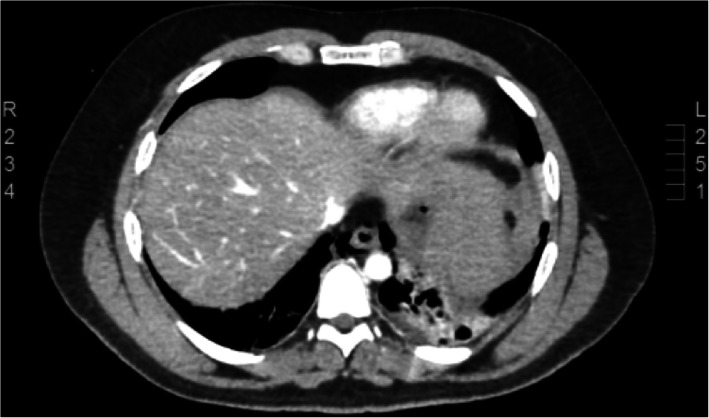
A CT Abdomen and pelvis, showed esophageal rupture at the gastro-esophageal junction with fundal intramural hematoma
